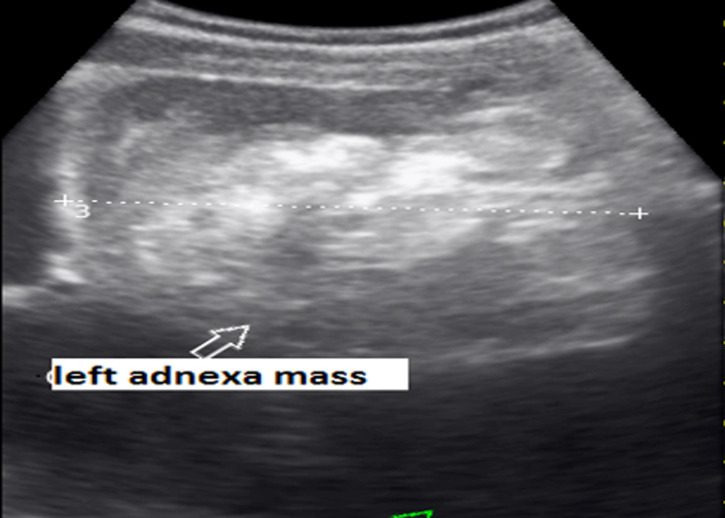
ultrasound image showing a complex left adnexal mass 12 cm x 10 cm
